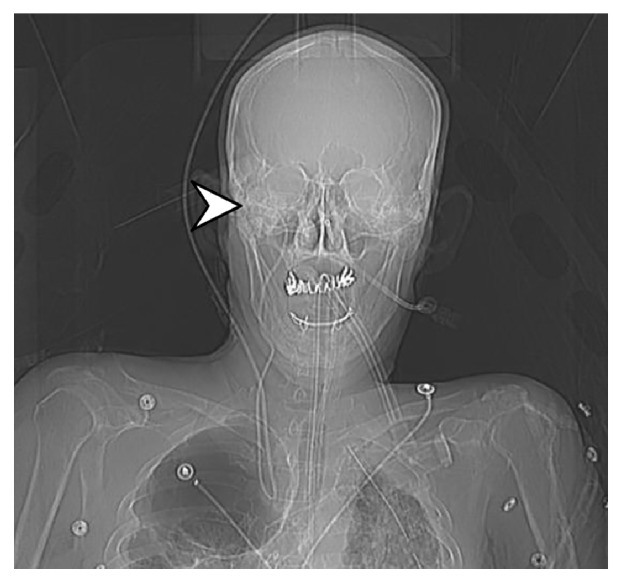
CT Venogram of the head with arrow depicting mispositioned tip of the venous catheter within the right internal jugular vein at the level of the jugular foramen.
